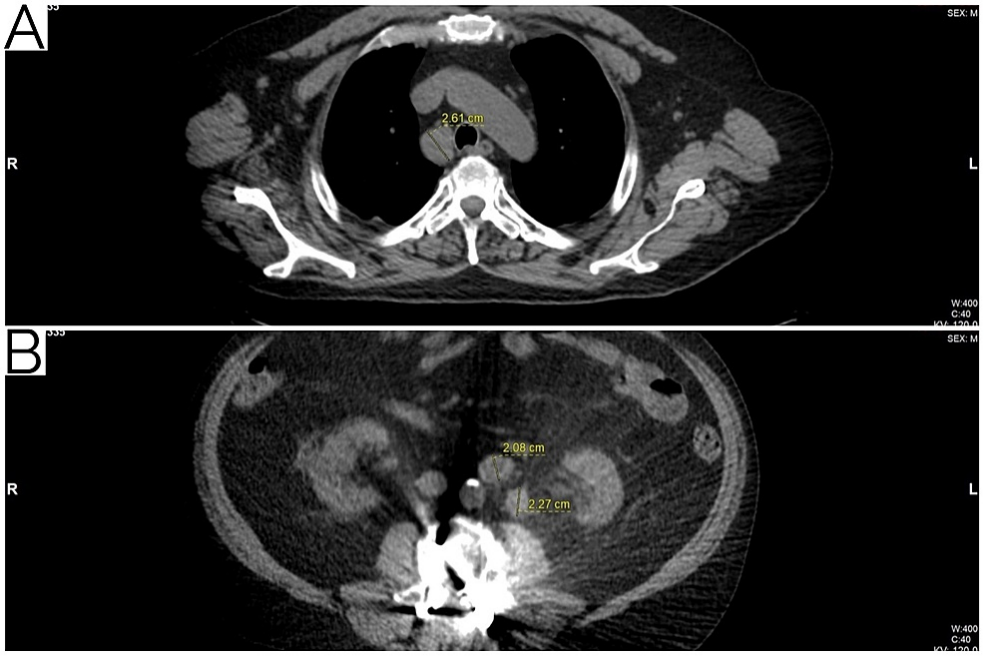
Post-treatment radiology. Post-treatment radiology of pathologic lesions, (A) right paratracheal mass and (B) left retroperitoneal mass, on CT correlates of PET. CT, computed tomography; PET, positron emission tomography.
